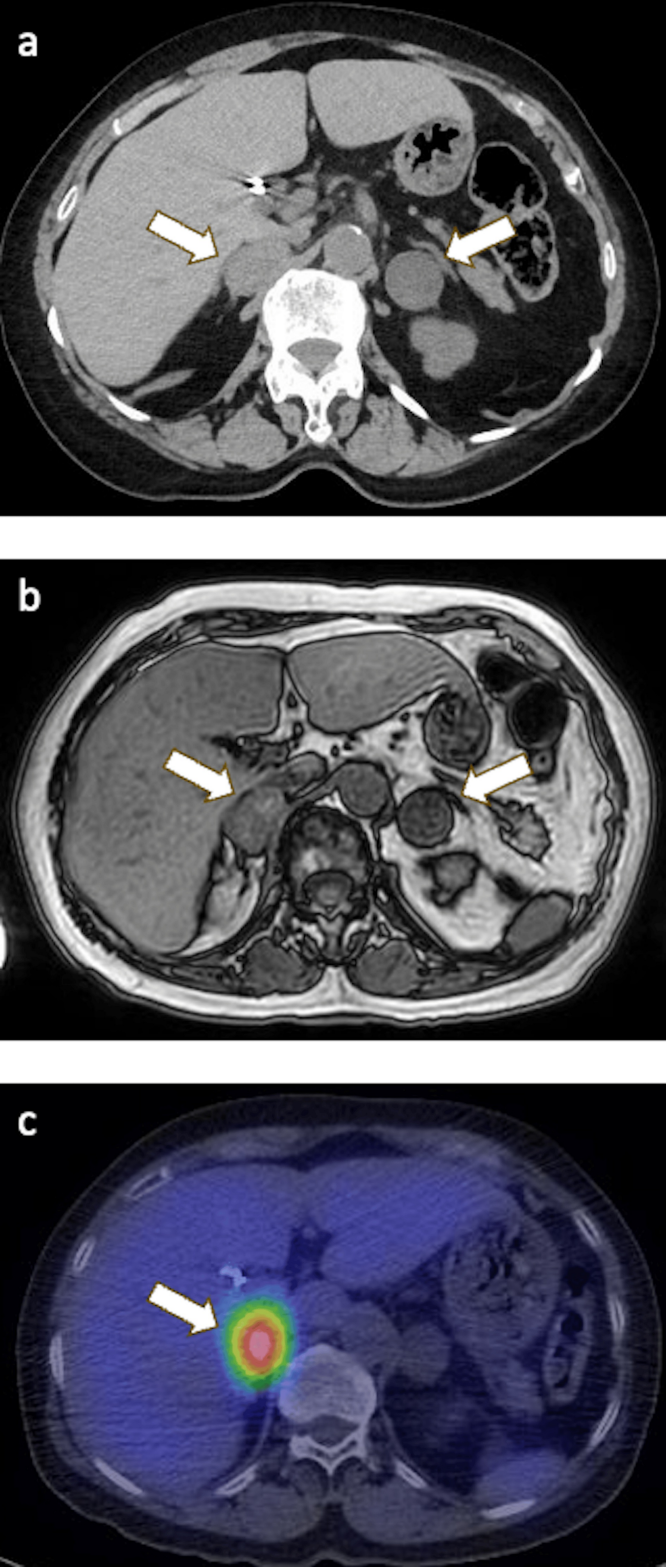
Adrenal lesions. (a) Abdominal CT showing a 32 mm right adrenal mass and a 26 mm left adrenal mass. (b) MRI depicting both adrenal masses. (c) MIBG scintigraphy revealing strong uptake in the right adrenal mass, with no uptake in the left. Arrows indicate each lesion.
